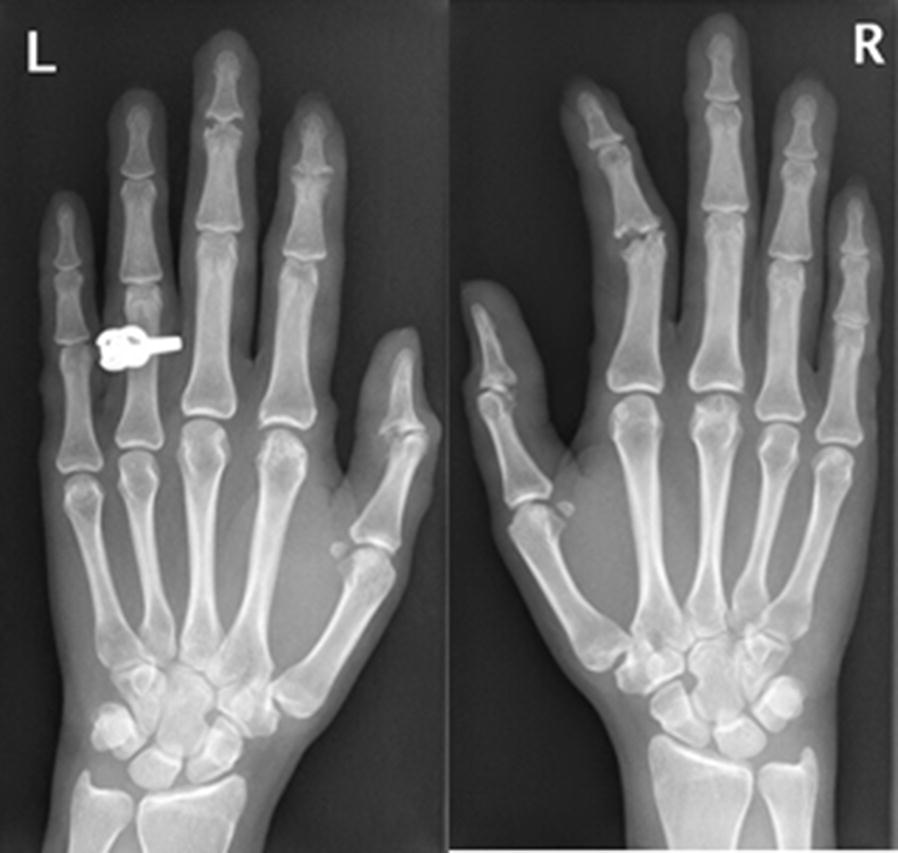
Radiographs of the hands, showing impressive erosions and destruction of small joints
